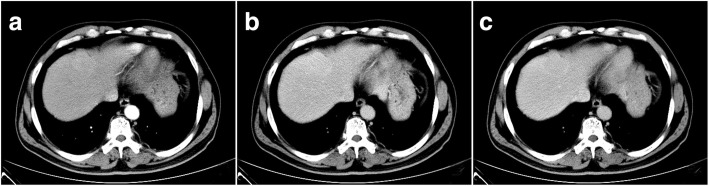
Contrast-enhanced computed tomography scan of intrahepatic splenosis. The lesion had an inhomogeneous enhancement during the arterial phase (a) and diminished enhancement during the portal phase (b) and equilibrium phase(c)
